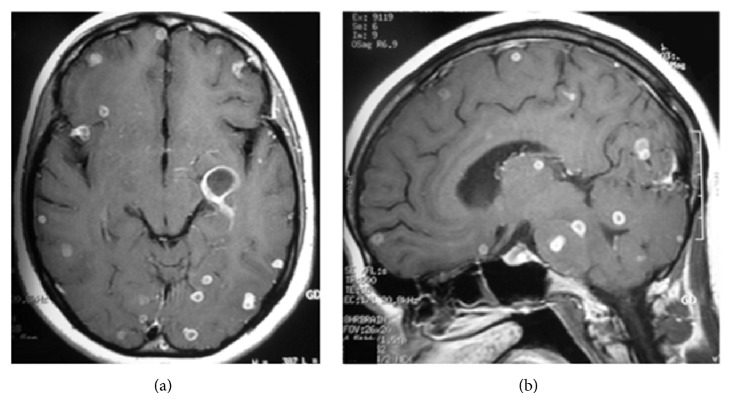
Multiple supra- and infratentorial tuberculomas in a 27-year-old female with history of pulmonary tuberculosis. Tuberculomas are seen as multiple small ring enhancing lesions without peripheral edema in axial and sagittal postcontrast T1-weighted MR images.
